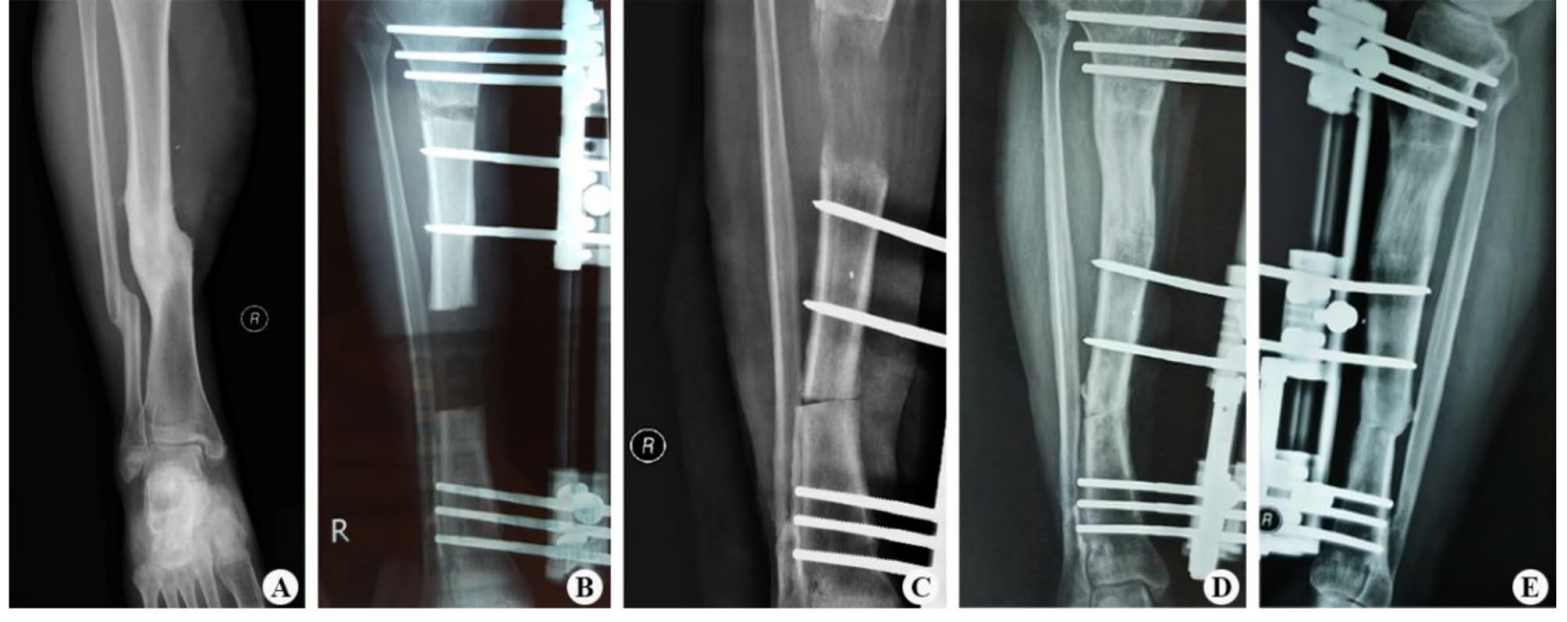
A 39-year-old male with nonunion of the right tibia caused by FRI was treated with single-level bone transport. (A) Nonunion of the right tibia caused by FRI. (B) X-ray showed that DS after debridement was almost 5.3 cm at the intermediate part of the tibia. (C) Third postoperative weeks at the distraction phase. (D, E) Bone transport was completed with a satisfactory bone union after 6 postoperative months
